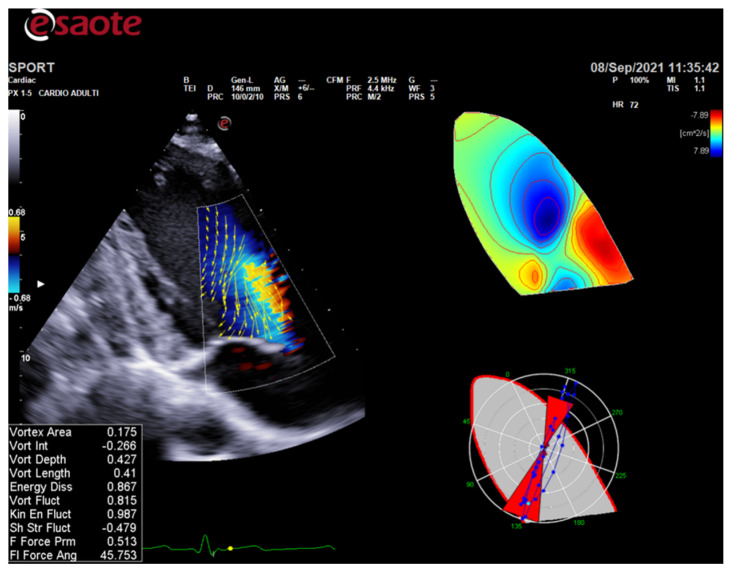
HyperDoppler flow analysis in an endurance athlete. On the left, the flow velocity vector map shows the blood flow that circulates towards the direction of the left ventricular outflow tract. On the right, the circulation parametric map brings to light the formation of a pair of vortices immediately below the aortic valve.
